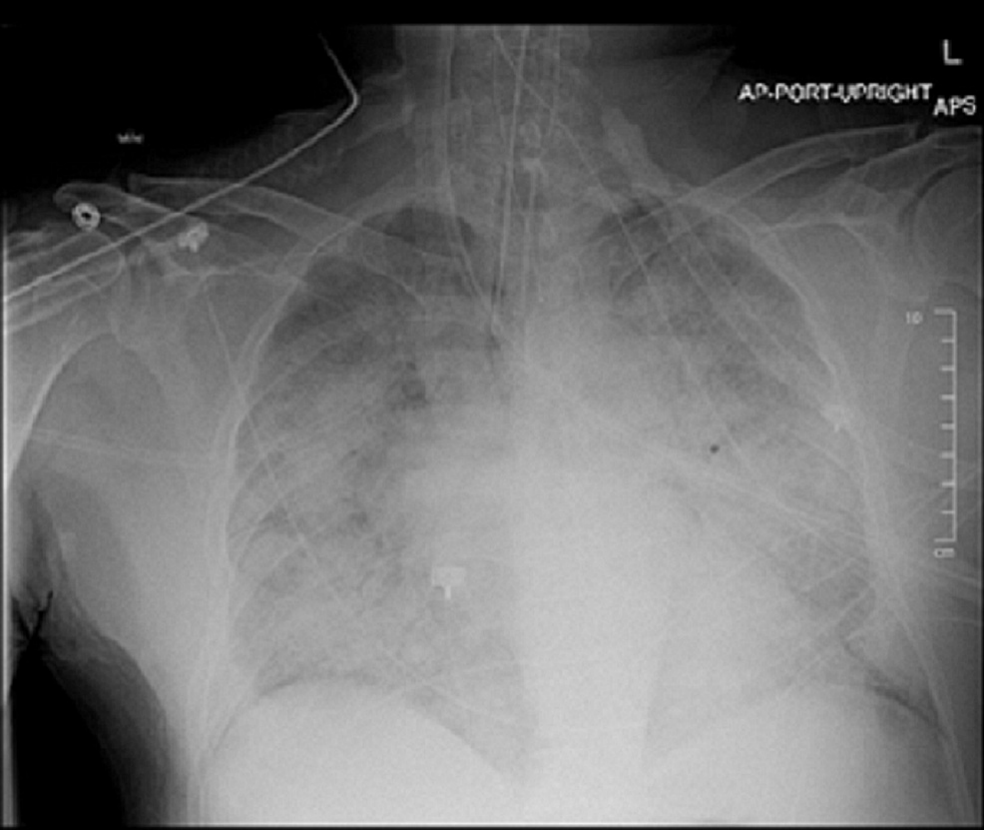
Chest X-ray showing diffuse interstitial and patchy alveolar opacities
